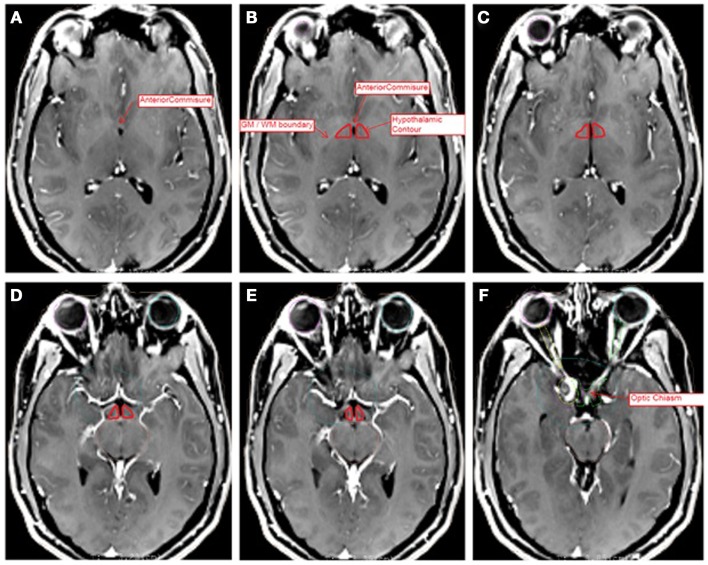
Representative slices of MRI T1 + C images registered to CT data sets for hypothalamic volume contouring. Slices A (superior most) through F (inferior most) depict several anatomic landmarks for the delineation of the hypothalamic contour (red). (A) Depicts the superior most slice, at the level of the anterior commissure. (B–E) Depict the hypothalamic contour, bounded by the white matter tracts laterally, the third ventricle medially, the CSF space of the suprasellar cistern anteriorly, and the level of the interpeduncular fossa posteriorly. (F) Depicts the level of the optic chiasm, at which the contour ends. The contour has been enhanced for ease of visibility.
